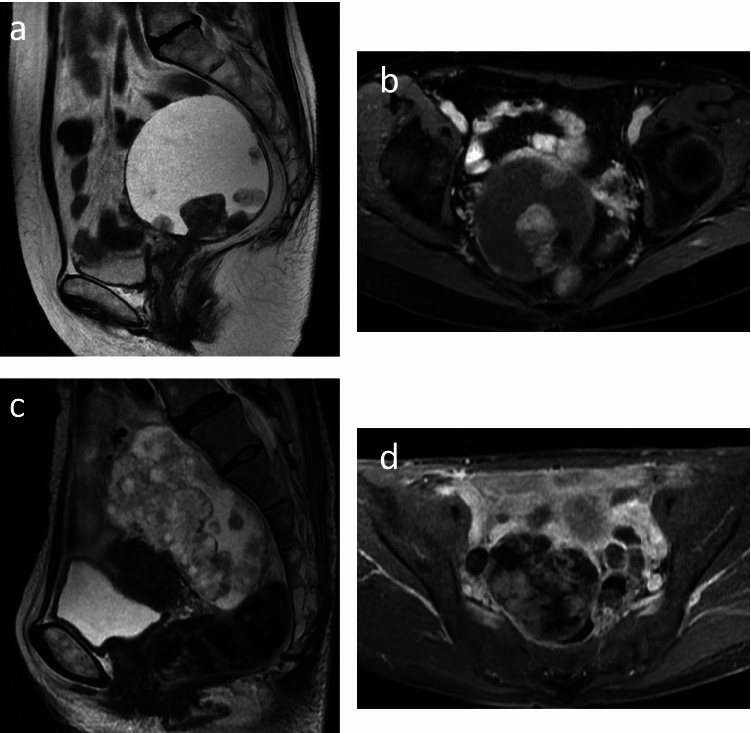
Growth pattern of the MRI-based features in the solid component of ovarian carcinomas. a, b Polypoid, focal, or eccentric growth pattern of the solid component without continuity in clear cell carcinoma on fast-spin echo (FSE) T2-weighted image (T2WI) and contrast-enhanced T1-weighted image (T1WI). c, d Broad-based, multifocal, or concentric growth pattern of the solid component with continuity in clear cell carcinoma on FSE T2WI and contrast-enhanced T1WI
